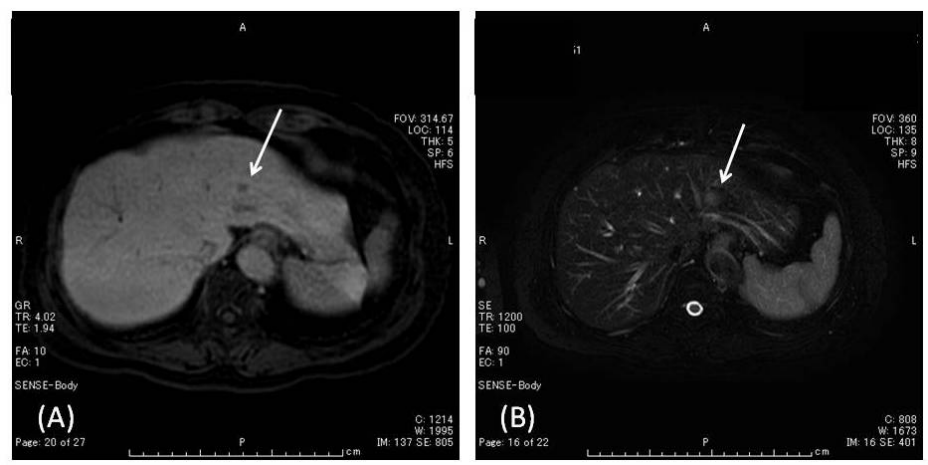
Magnetic resonance imaging (MRI), the hepatic tumor (arrow) was low signal intensity in T1-weighted image (A) and slight high signal intensity in T2-weighted image (B), and low signal intensity in hepatobiliary phase after Gd-EOB-DTPA injection, and on dynamic Gd-EOB-DTPA MRI protocol not clearly visualized during arterial dominant phase with slight ring-like enhancement persisting, indicating hypovascular tumor, such as cholangiocarcinoma or liver metastasis.
